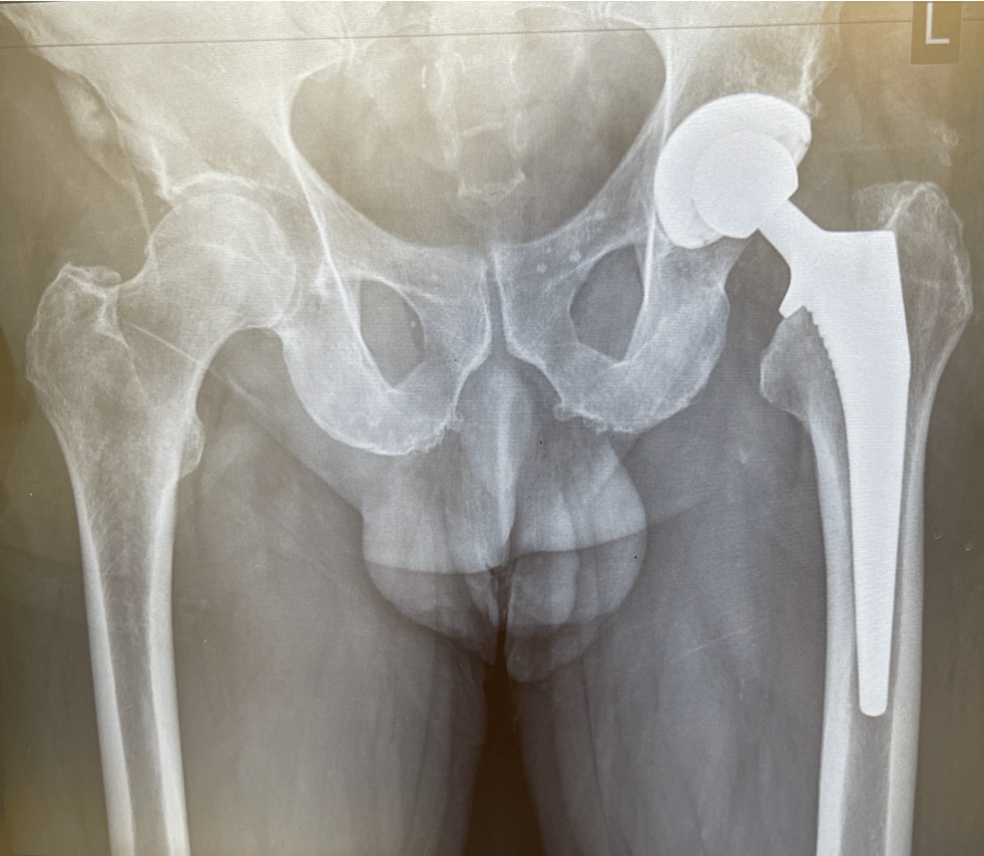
Example of uncemented collared pinnacle/corail THR, with >2 mm lucency in zone 1 of the femoral stem (12 months post-operative radiograph).
